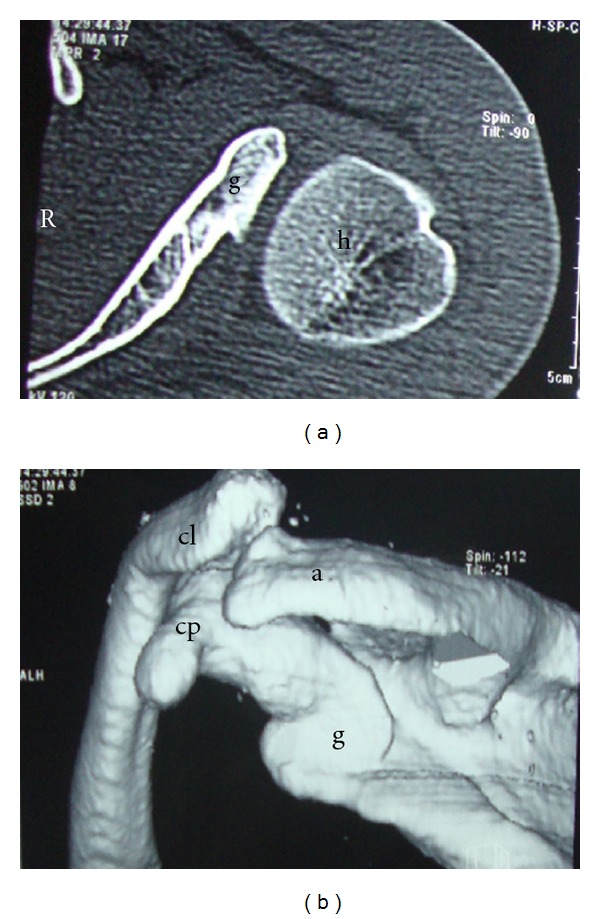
(a) CT scan showing (g) hypoplastic glenoid and (h) normal humeral head. (b) Three-dimensional CT scan showing (g) hypoplastic glenoid, (cp) prominent coracoid process, (cl) hooking of distal aspect of clavicle, and (a) prominent acromion.
